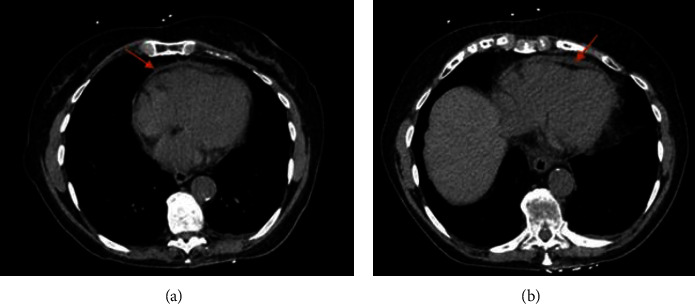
Computed tomography angiography with intravenous contrast of the chest demonstrating trace pericardial effusion (red arrows).
